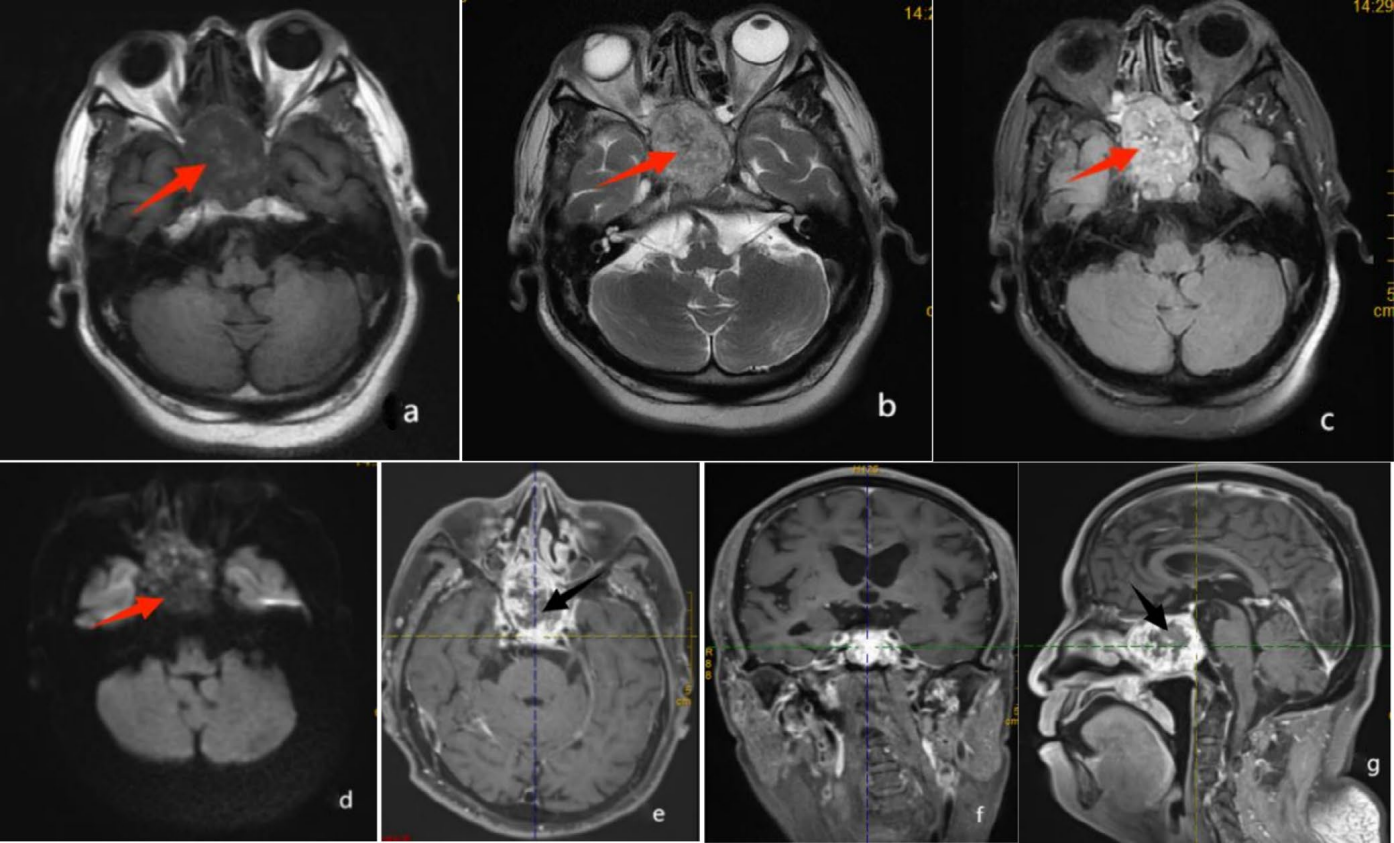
Plain and enhanced magnetic resonance images of the patient with a mass shadow in the sphenoid sinus region. (a) T1‐weighted imaging showing an equal and slightly low signal, with a few slightly high‐signal shadows in the center (red arrow). (b) T2‐weighted imaging and (c) T2FLAIR imaging showing a mixed, slightly high signal (red arrow). (d) Diffusion‐weighted imaging showing the mass as mostly isointense, with a few localized spots of slightly high signal (red arrow). (e–g) Enhancement images showing obvious uneven enhancement, with a patchy, non‐enhanced area in the center (black arrow), and the mass is not clearly demarcated from the pituitary gland.
